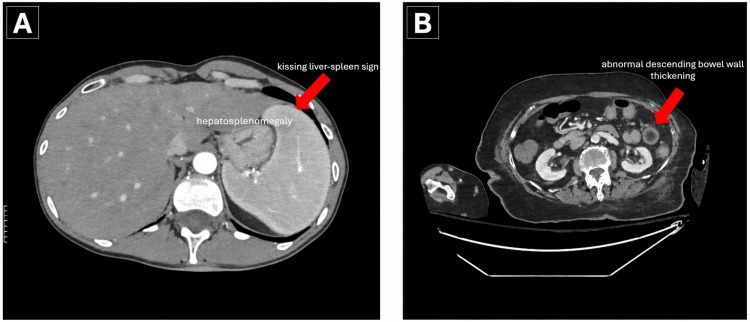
Contrast-enhanced axial abdominal CT showing hepatosplenomegaly (A) and colitis (B) (A) Marked hepatosplenomegaly with close apposition of the enlarged liver and spleen, producing the “kissing liver–spleen” sign; the red arrow indicates the area of liver–spleen contact. (B) Segmental abnormal wall thickening of the descending colon, compatible with colitis; the red arrow indicates the affected colonic segment CT: computed tomography
